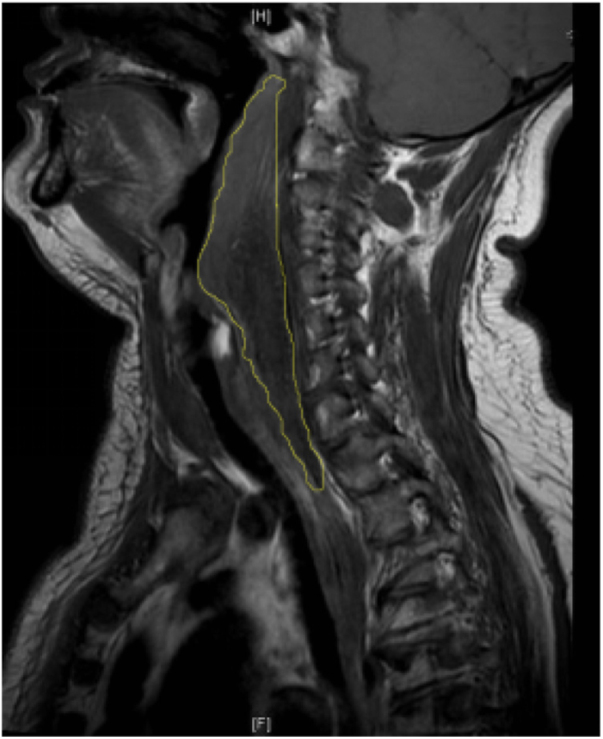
Sagittal magnetic resonance imagery revealed an abnormal irregular signal extending from the second cervical vertebra to the posterior mediastinum.
